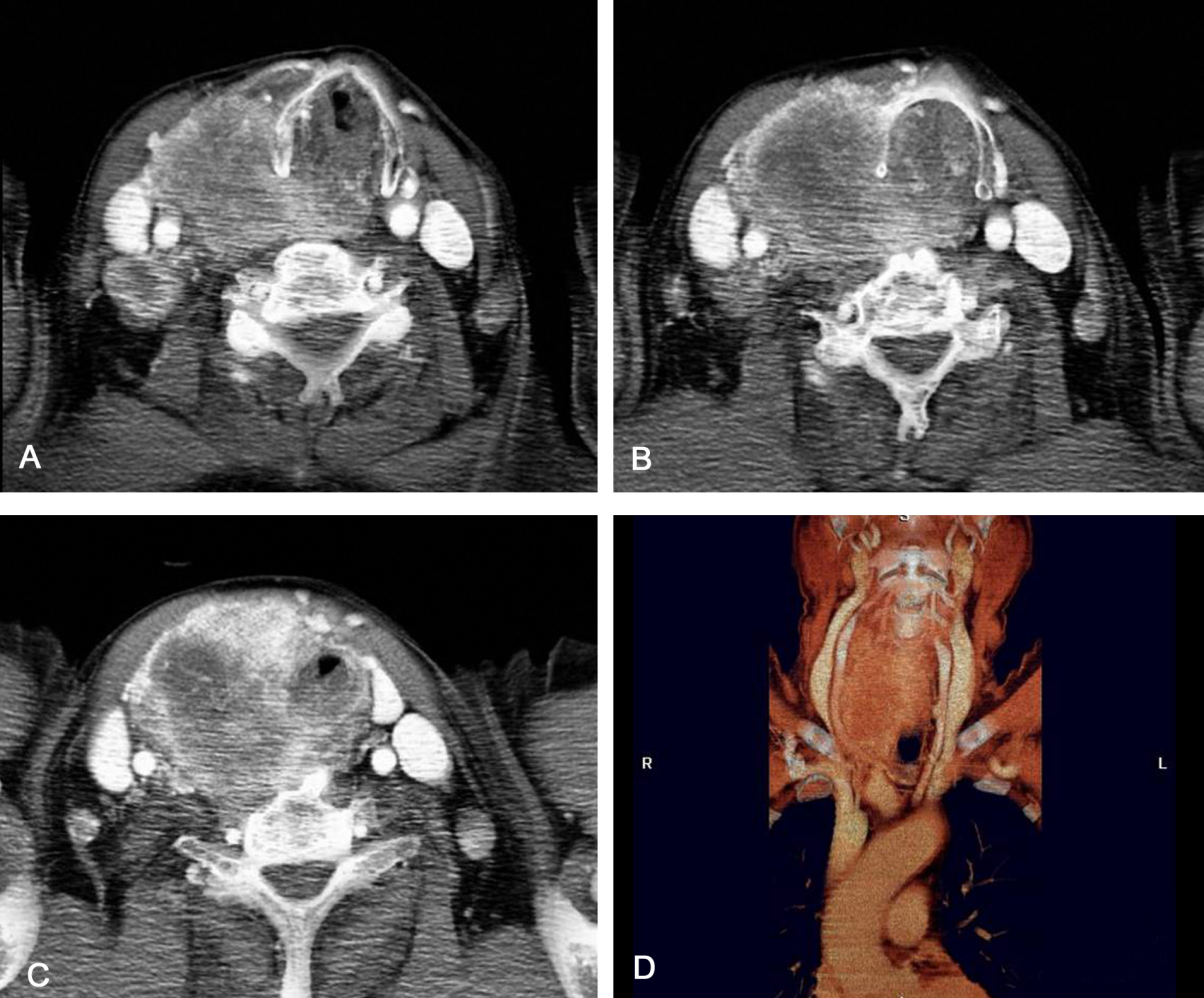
Contrast enhanced CT scan, axial images and coronal reconstructed image. Axial images sequences show the complete closure of the tracheal lumen. A thyroid mass with substernal extension, and with right jugular vein and carotid artery compression and displacement, in addition to diffuse lymphadenopathy are also evident.
